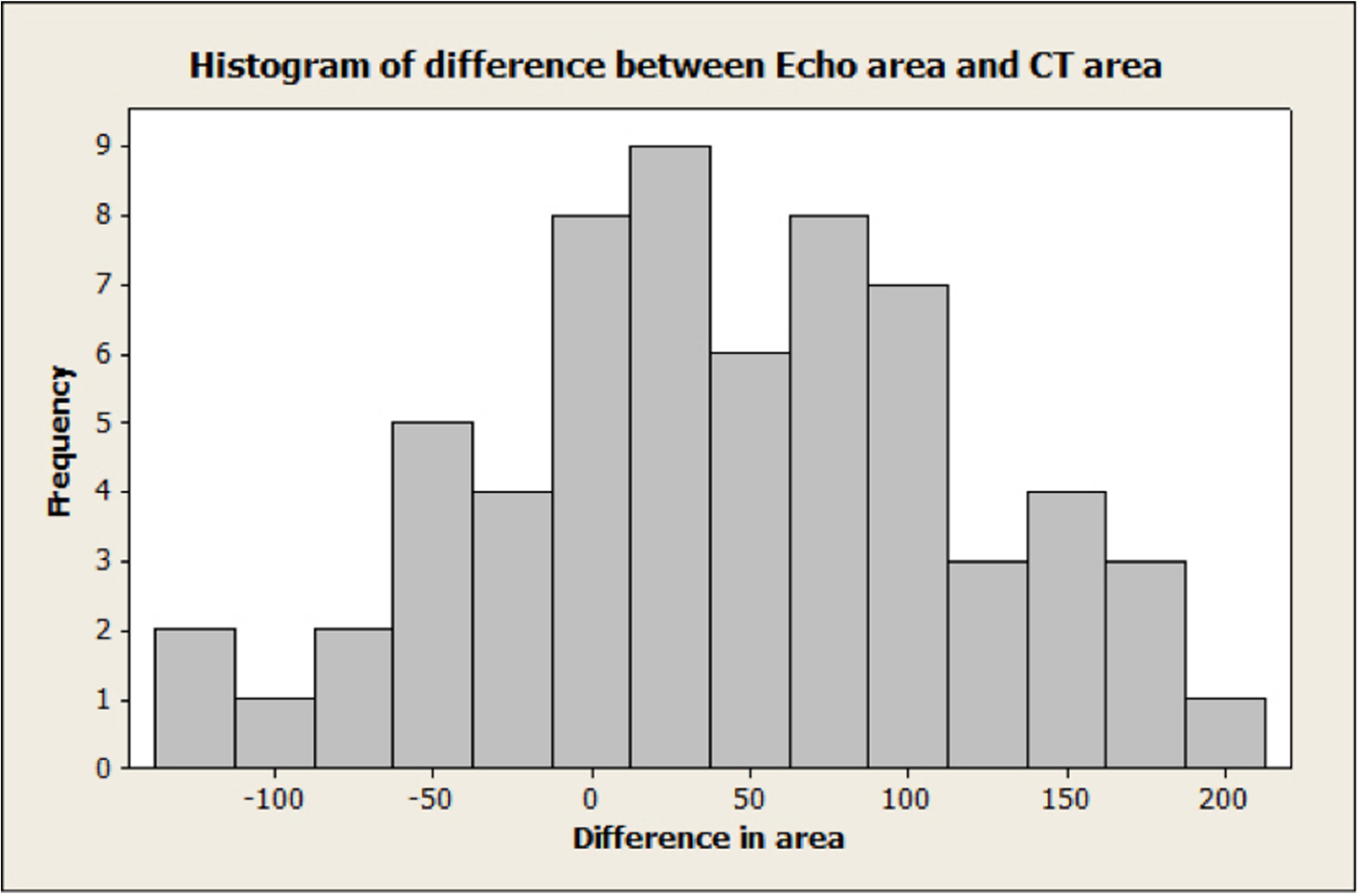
A histogram of the difference: TEE derived area minus CT derived area. There is a tendency for the echo areas to be higher (*p* < 0.001, *t*-test)
